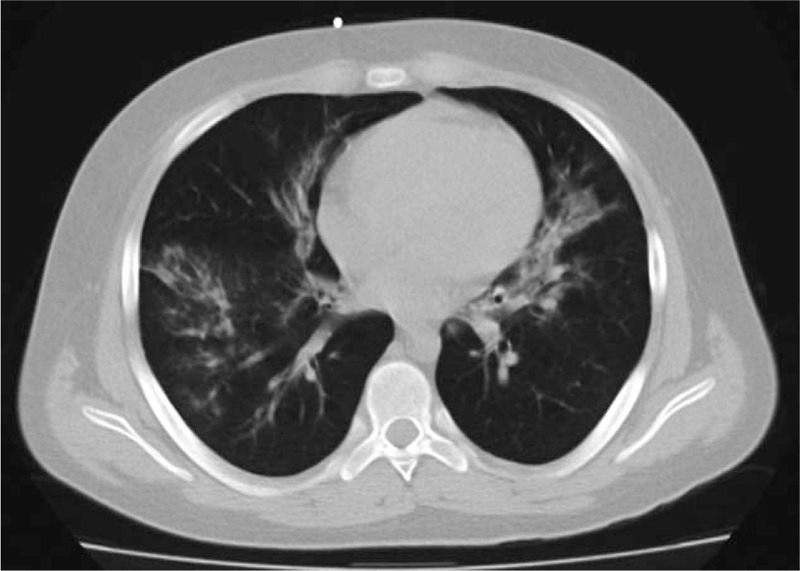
Computed tomography of the chest of the second child when clinical symptoms appeared.
